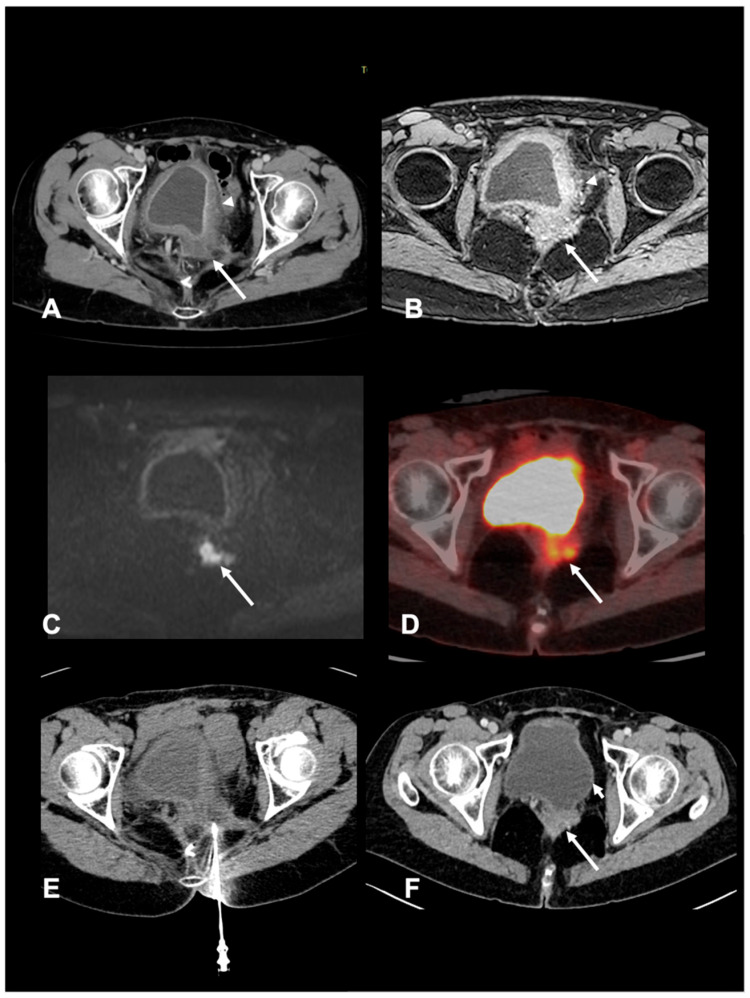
Multimodal imaging, image-guided biopsy, and radiological response after medical therapy of rectovesical xanthogranulomatous inflammatory mass. Panel legend. (A) CT of the pelvis demonstrated parietal thickening of the left wall (arrowhead) and posterior wall (arrow) of the bladder with a solid mass approximately 41 × 26 mm. The edges of the tissue were blurred and a peripheral rim enhancement was observed. (B) MRI of the pelvis confirmed and better depicted the pathological tissue (arrow), with more evident contrast enhancement compared with CT. Diffusion-weighted imaging (DWI) evidenced high cellularity (arrow). (C) Diffusion-weighted imaging (DWI) evidenced high cellularity (arrow). (D) PET-CT highlighted FDG uptake of the nodular portion of the pathological tissue, with very good agreement with DWI findings (arrow). (E) CT-guided biopsy with an 18G cutting needle of the nodular component of the lesion (arrow). (F) Follow-up CT after 6 months of therapy demonstrated a marked reduction in pathological tissue size, with normalization of the left bladder wall (arrowhead) and shrinkage of the posterior nodular component to approximately 27 × 17 mm (arrow).
